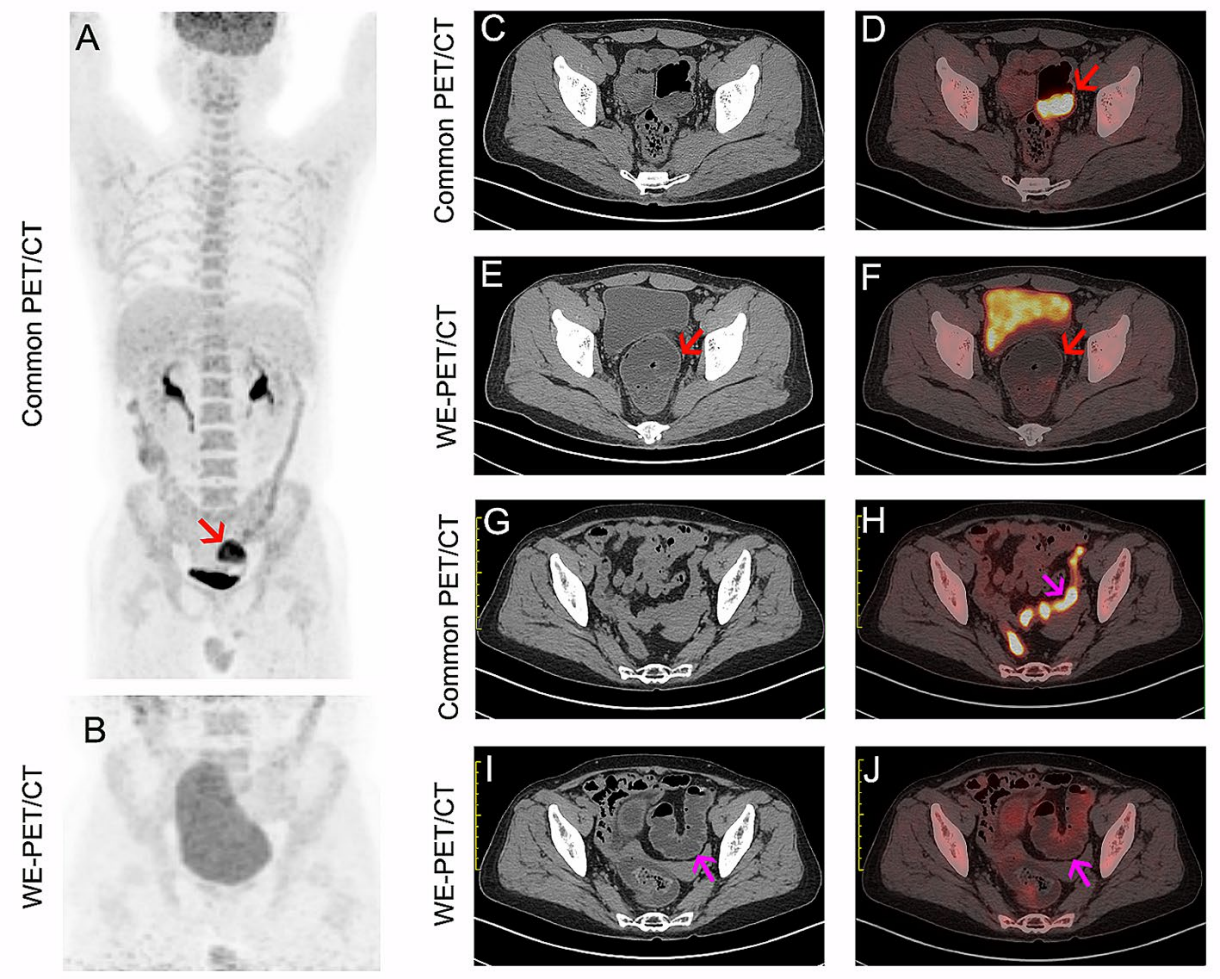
Representative image of focal (A-F, red arrow) and segmental (G-J, pink arrow) physiologic ^18^F-FDG uptake. The ^18^F-FDG uptake on common PET/CT (A, D and H) disappeared after water enema (B, F and J)
